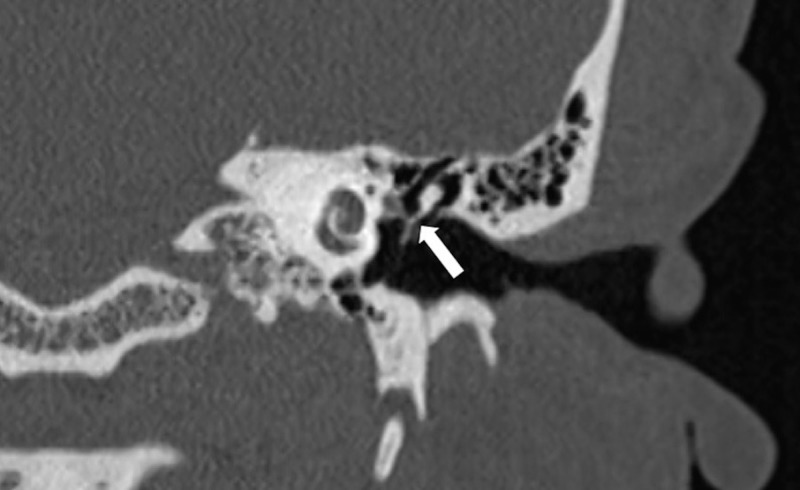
Preoperative Computed Tomography of the Left Temporal Bone Preoperative high-resolution computed tomography scan of the left temporal bone in the coronal plane demonstrating a nondisplaced transverse lucency of the malleus (white arrow).
